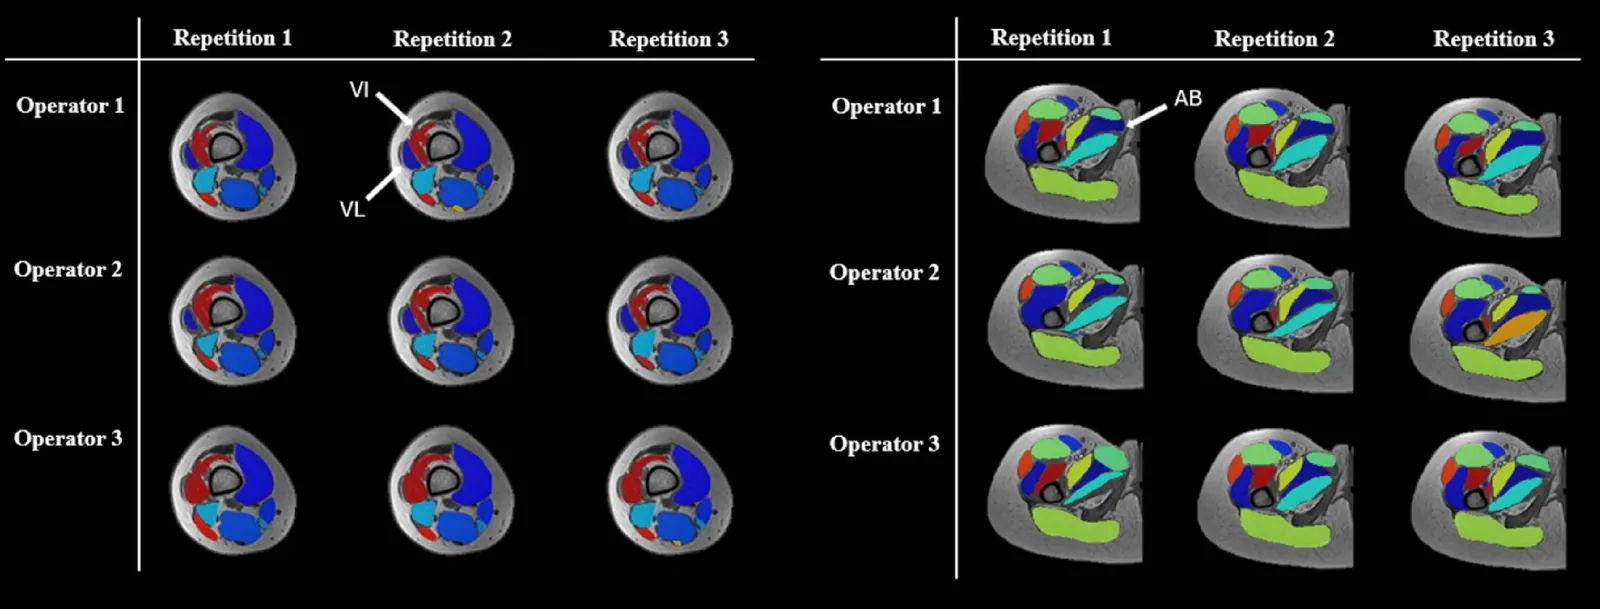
Visualization of manual segmentation across 3 repetitions among 3 operators. The label combined MR images in the upper (right, close to hip part) and lower (left, located at the mid-thigh) sections are from the same subject selected for the repeatability analysis. Repetition 1–3 corresponds to the first through the last trial segments performed by the same operator.
